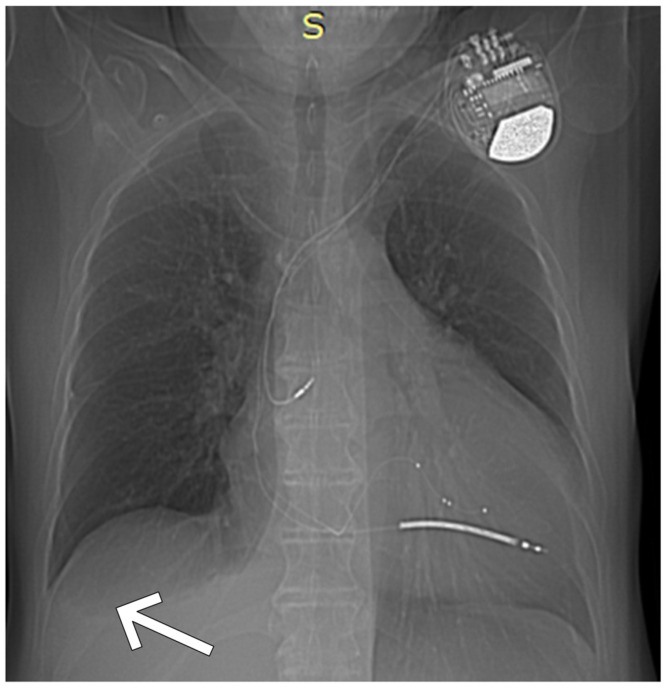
Chest X-ray: enlarged cardiac silhouette and blunting of the right costophrenic angle with pleural effusion. The white arrow indicates the area of effusion.
